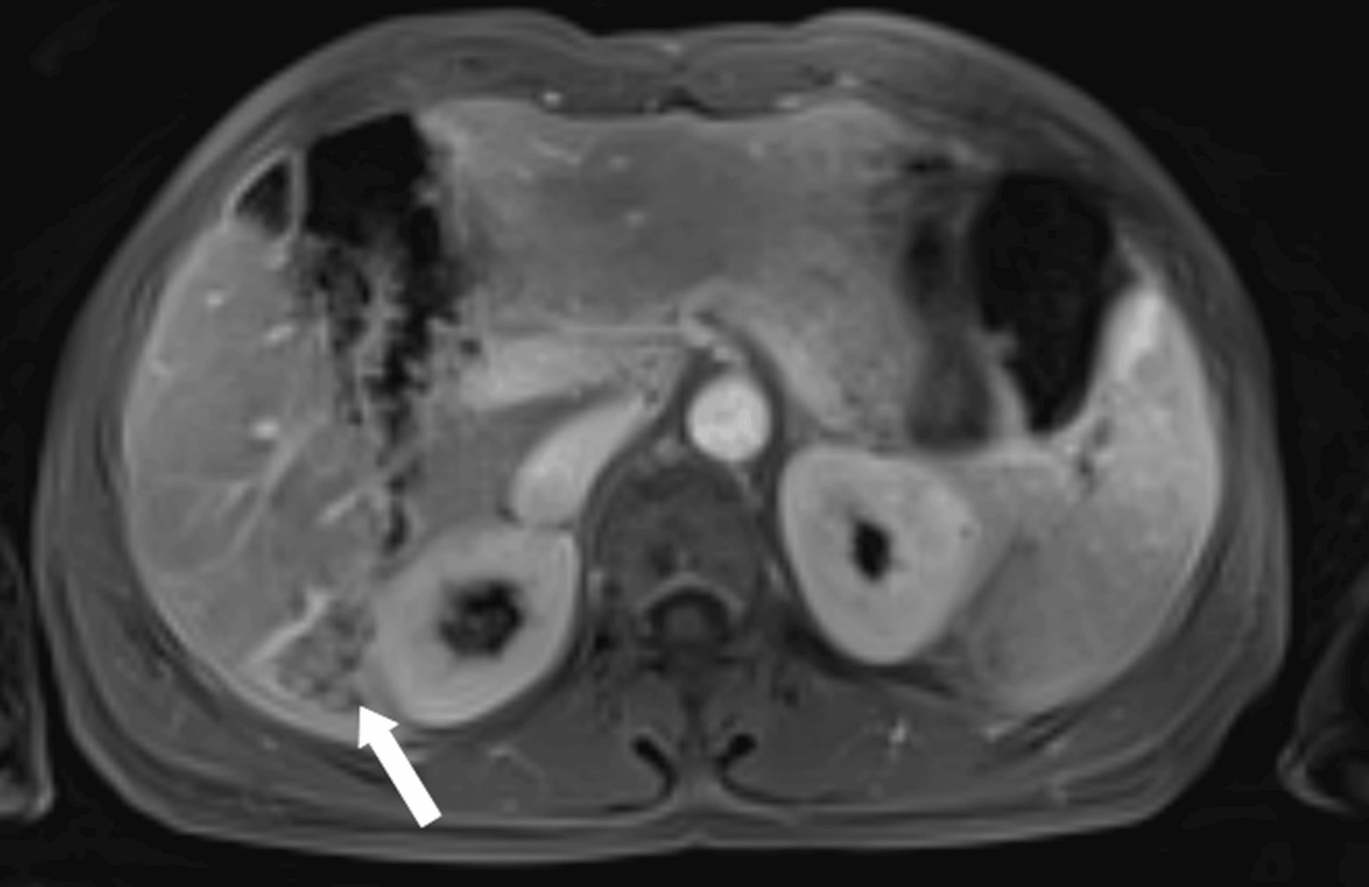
Axial contrast-enhanced MRI in the late venous phase at the level of the lesion Late venous phase MRI demonstrating the lesion becoming isotense to slightly hypointense relative to the liver parenchyma (white arrow).
